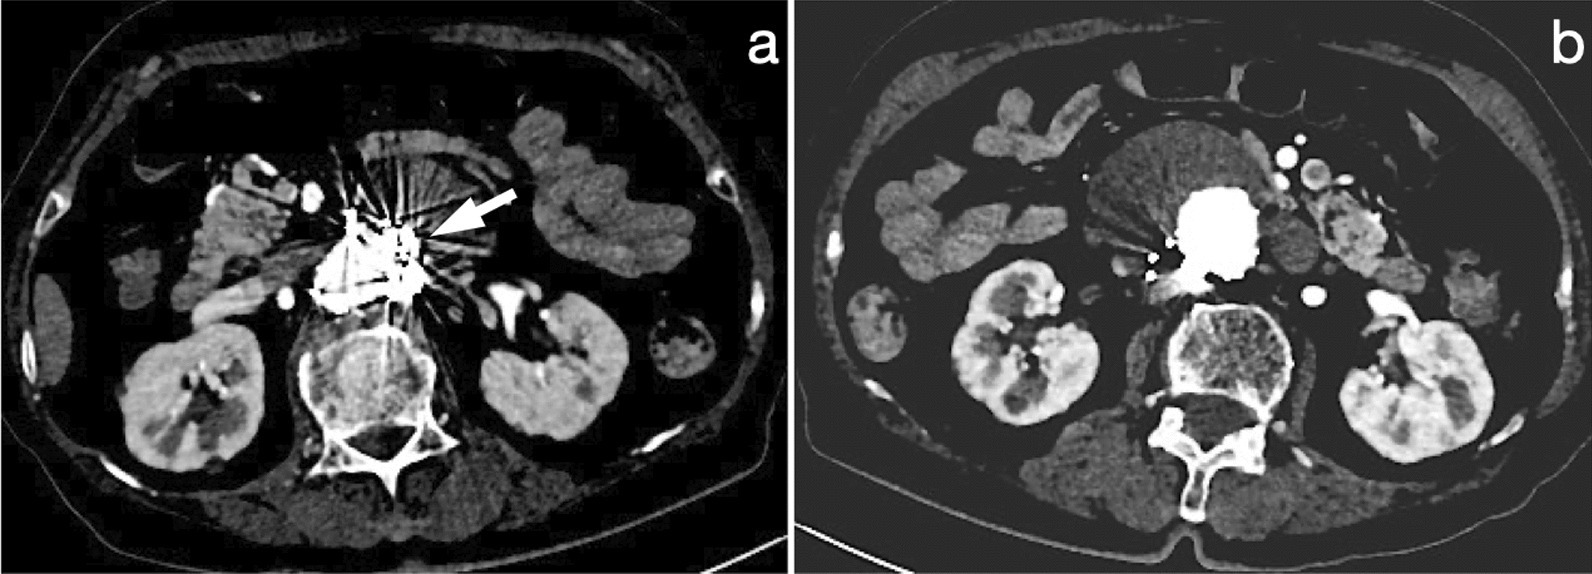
CTA during follow-up. a CTA 1 year after EVAR revealed no endoleak, and coils (white arrow) were placed around the sac neck. b There was no endoleak, and a sac regression of 7 mm was observed
